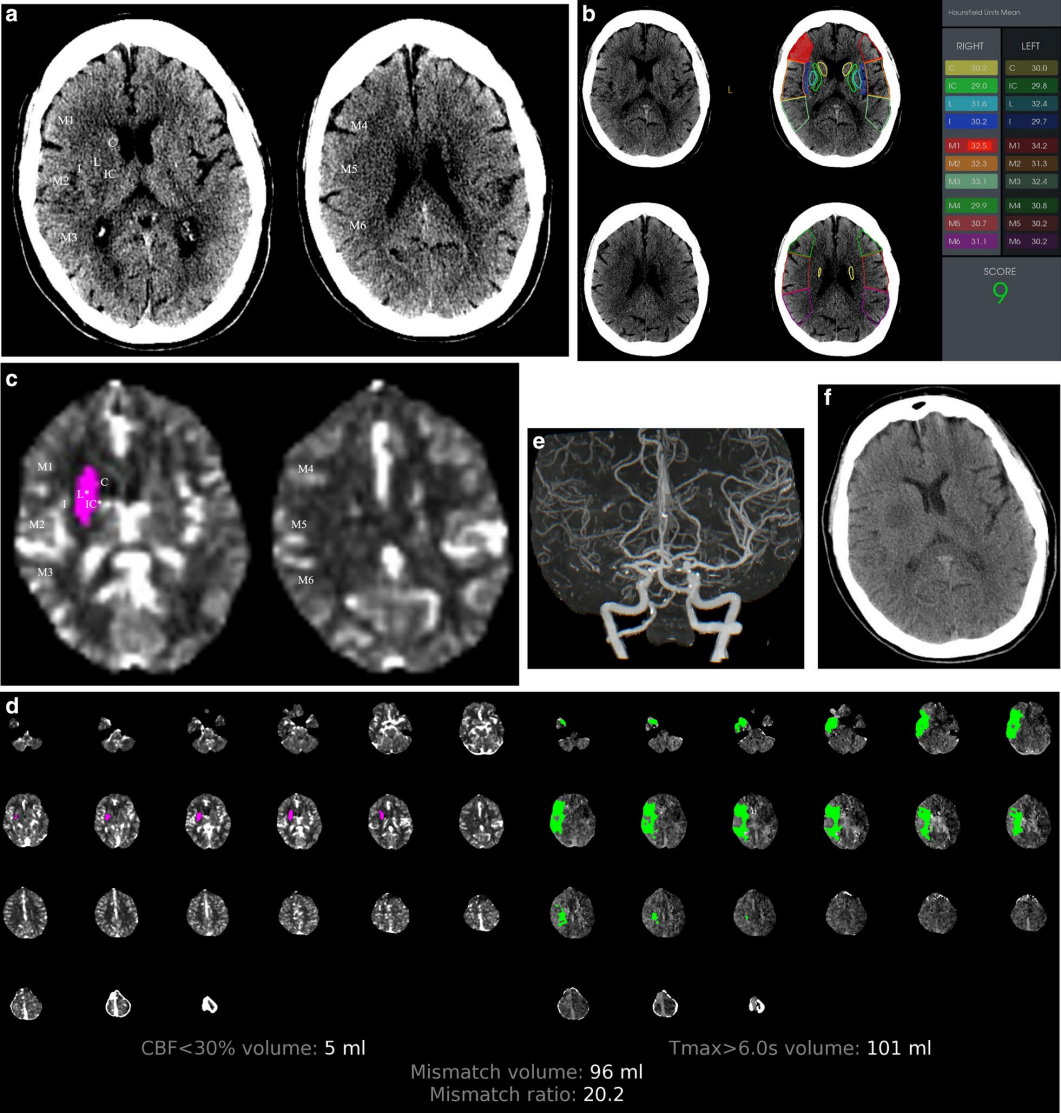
An 80-year-old female patient arrived at the hospital 1.5 h after the onset of symptoms, with a baseline NIHSS score of 20. The NCCT-, Auto- and CTP-ASPECTS were 10, 9, and 8 points (a–c), respectively. CT perfusion showed an infarct core of 5 ml, hypoperfusion of 101 ml, mismatch volume of 96 ml, and mismatch ratio of 20.2 (d). The Auto-ASPECT showed ischemia in the M1 region, while the CTP-ASPECT showed ischemia in the internal capsule and the lentiform nucleus region. The maximum intensity projection showed that the proximal M1 segment of the middle cerebral artery was occluded (e). The infarct core shown by CT perfusion was equivalent to the final infarct volume shown by NCCT reviewed 16 h after successful mechanical thrombectomy (f). The patient had a good clinical outcome at the 90-day follow-up. * indicates the regions of the early ischemic changes of the 10 defined middle cerebral artery (MCA) vascular territories. C = caudate head, I = insula, IC = internal capsule, L = lentiform nucleus, M1 = frontal operculum, M2 = anterior temporal lobe, M3 = posterior temporal lobe, M4 = anterior MCA, M5 = lateral MCA, M6 = posterior MCA
